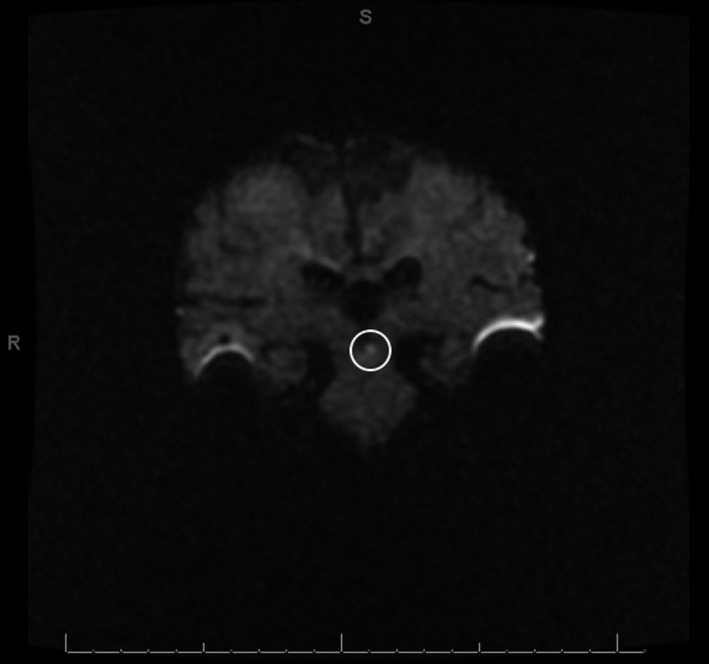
Hospital day 3 coronal DWI identifying the 3‐mm focus of restricted diffusion (circled) in the region of the left medial longitudinal fasciculus
